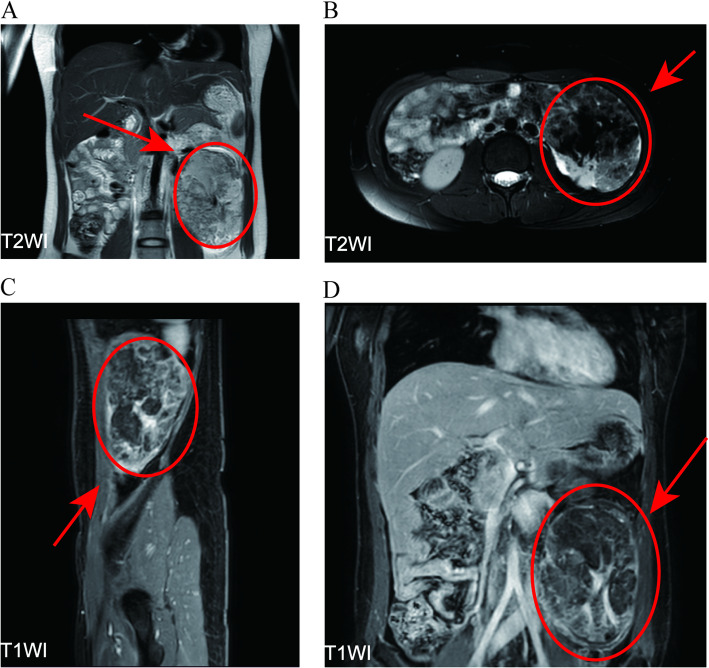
Pre-surgical MRI images. (A: T2WI, Frontal plane. B: T2WI, Axial section. C: T1WI, Sagittal plane. D: T1WI, Frontal plane)
